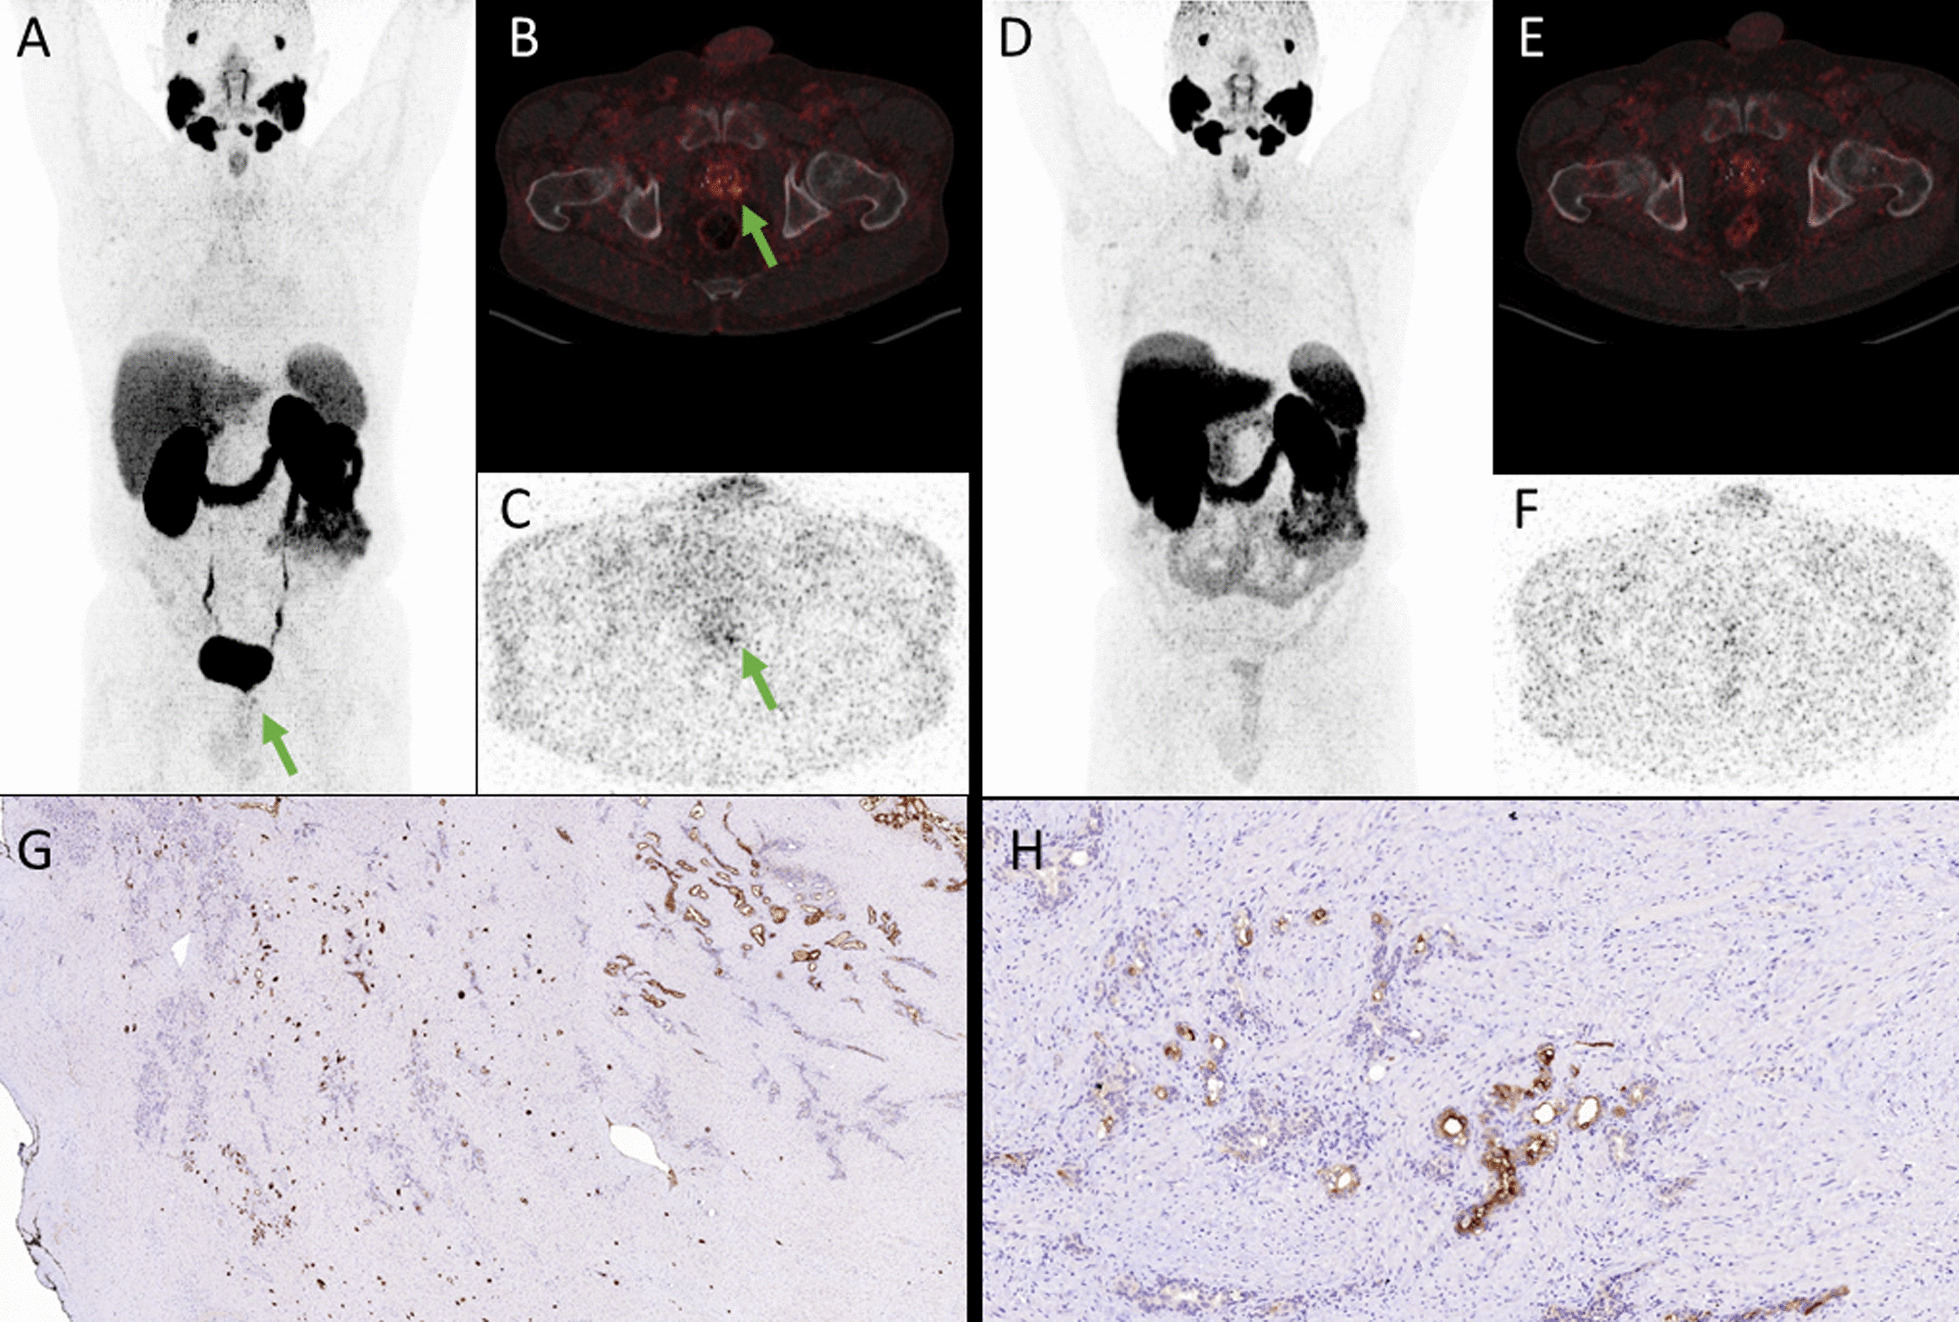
MIP of [^68^Ga]-Ga-PSMA-11 PET (A) with marginally positive (SUV_max_ 7.7) oligofocal local recurrence after seed implantation in both the right and left dorsoapical prostate lobe (green arrow). The accumulation was retrievable in both the attenuation corrected fused axial slices (B) and in the non-attenuation corrected axial slices (C). In [^18^F]-F-PSMA-1007 PET neither in the MIP (D) nor in the attenuation corrected fused axial slices (E) nor in the non-attenuation corrected axial slices (F) focal uptake was noted. Immunhistochemical images (G, H) show several, diffuse, small tumor cell nests with moderate to intense PSMA-expression
